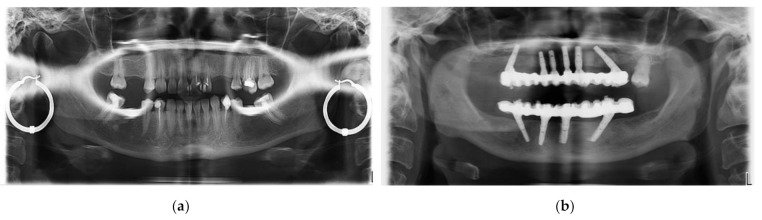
Imaging artefacts on panoramic radiographs: (a) in the case of a woman who cannot remove her earrings and (b) for a patient with ten implants and teeth reconstructions.
